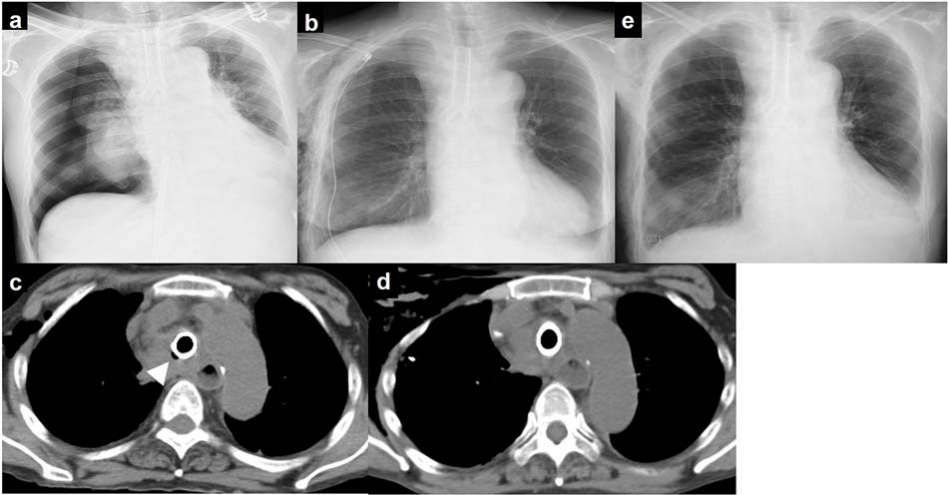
(a) chest X-ray showing right pneumothorax; (b) chest drainage was performed; (c) chest computed tomography immediately after surgery showing an air space on the right side of the stent; (d) the air space on the right side of the stent gradually disappeared; (e) chest X-ray showing no recurrence of pneumothorax.
